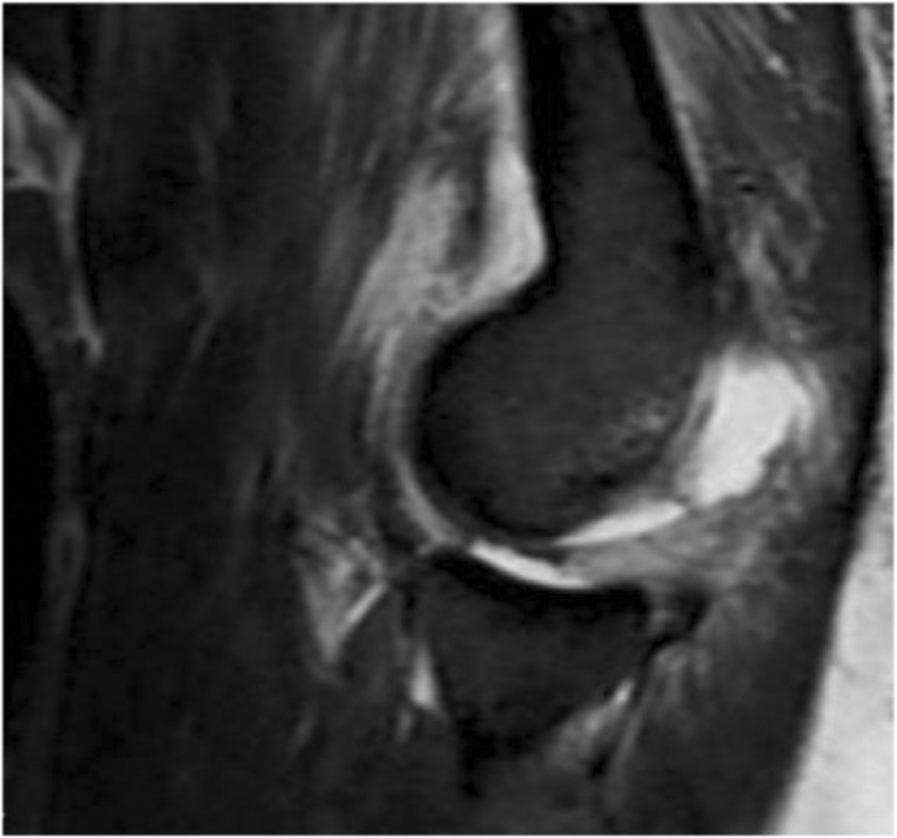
Complete tear of the LCL with posterior subluxation of the radial head in sagittal view in MRI
